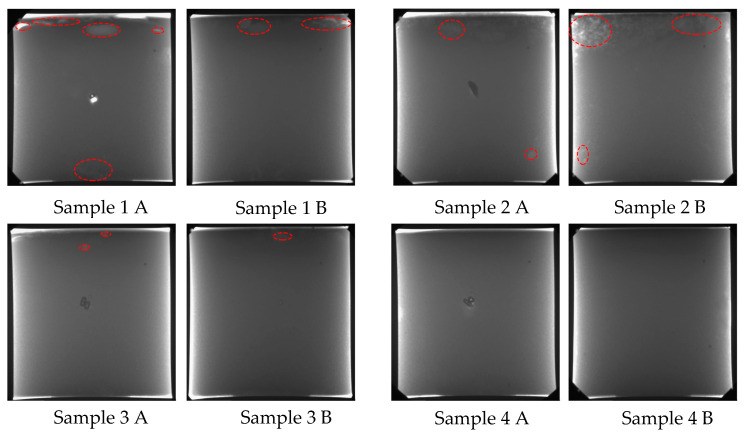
X-ray detection results of castings.
